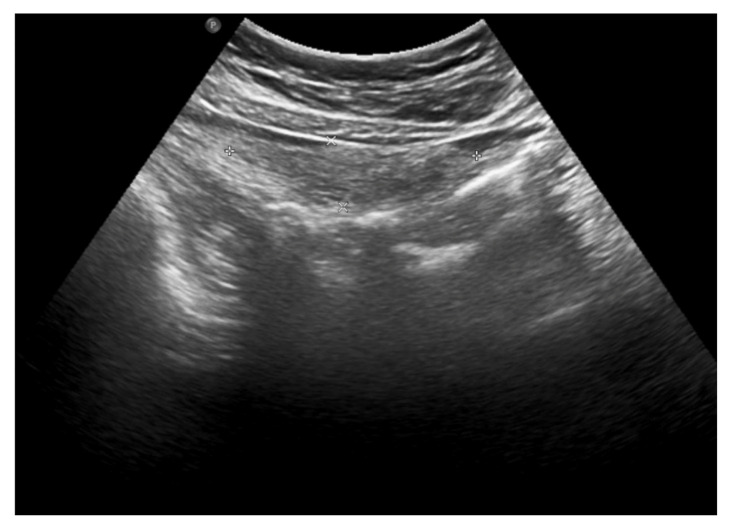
The abdominal ultrasound performed 2 weeks after hospitalization: further regression of abdominal lesions.
